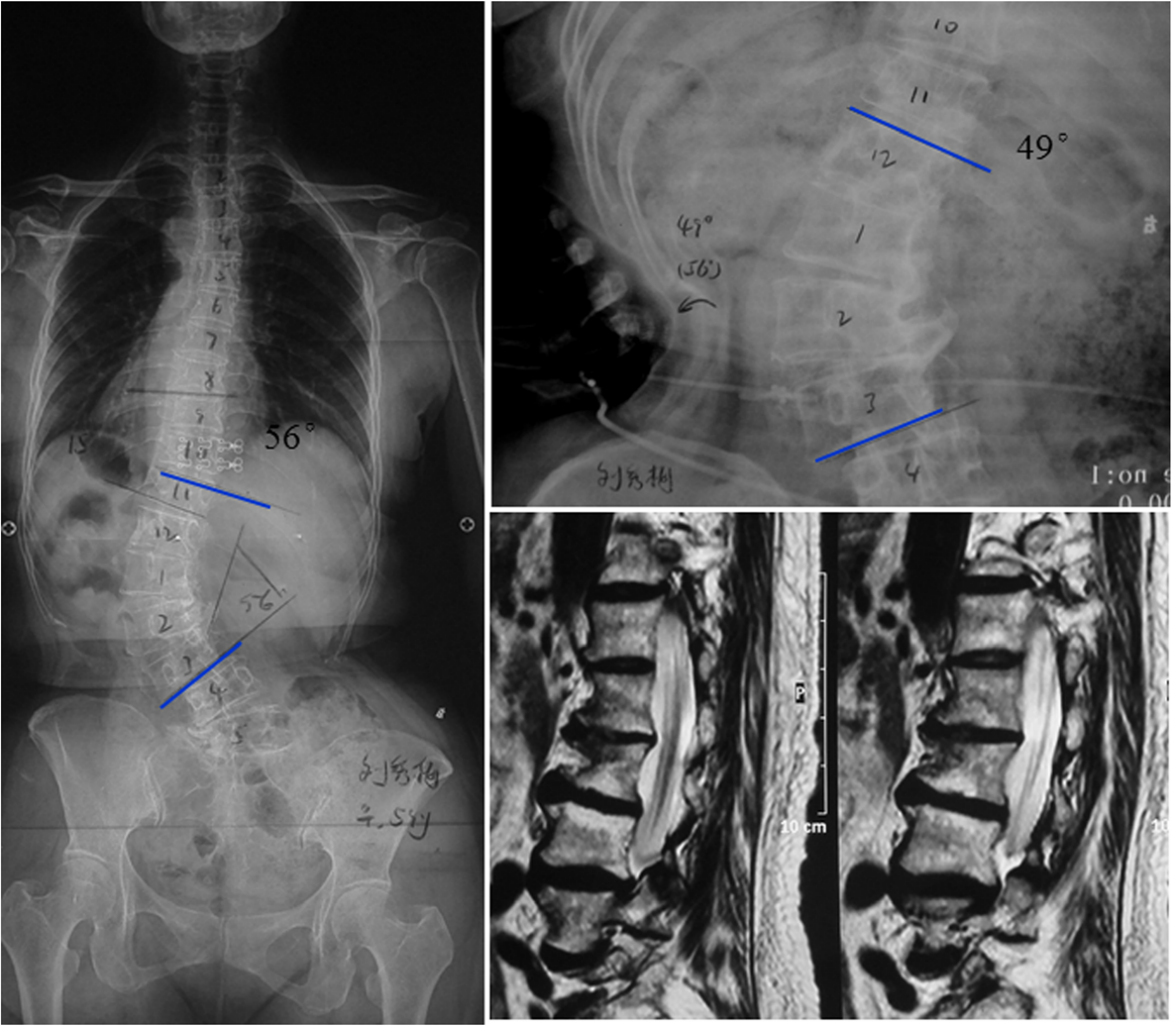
59 years old female patient with degenerative scoliosis. Lumbar Cobb angle was 56° on standing whole spine film. On supine lateral bending film, lumbar Cobb angle decreased to 49°, with a correction rate of only 12.5%. Lumbar MRI showed severe disc degeneration; Grade IV osteophyte could be found on concave side of the curvature
